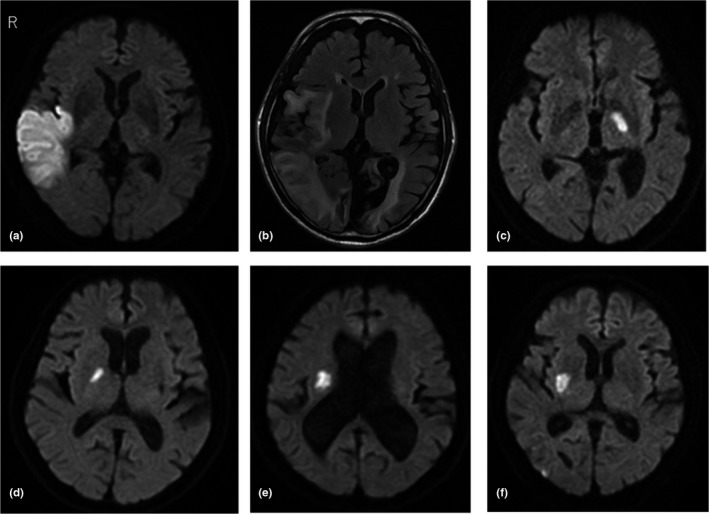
Radiological findings for patients with supratentorial infarcts. We could not obtain radiological data for Patient 1. (a) Diffusion‐weighted imaging (DWI) for Patient 2, obtained 4 days after onset. (b) Fluid‐attenuated inversion recovery imaging in Patient 3, obtained 6 days after onset. (c) DWI in Patient 4, obtained 3 days after onset. (d) DWI in Patient 5, obtained 3 days after onset. (e) DWI in Patient 6, obtained 4 days after onset. (f) DWI in Patient 7, obtained 1 day after onset
